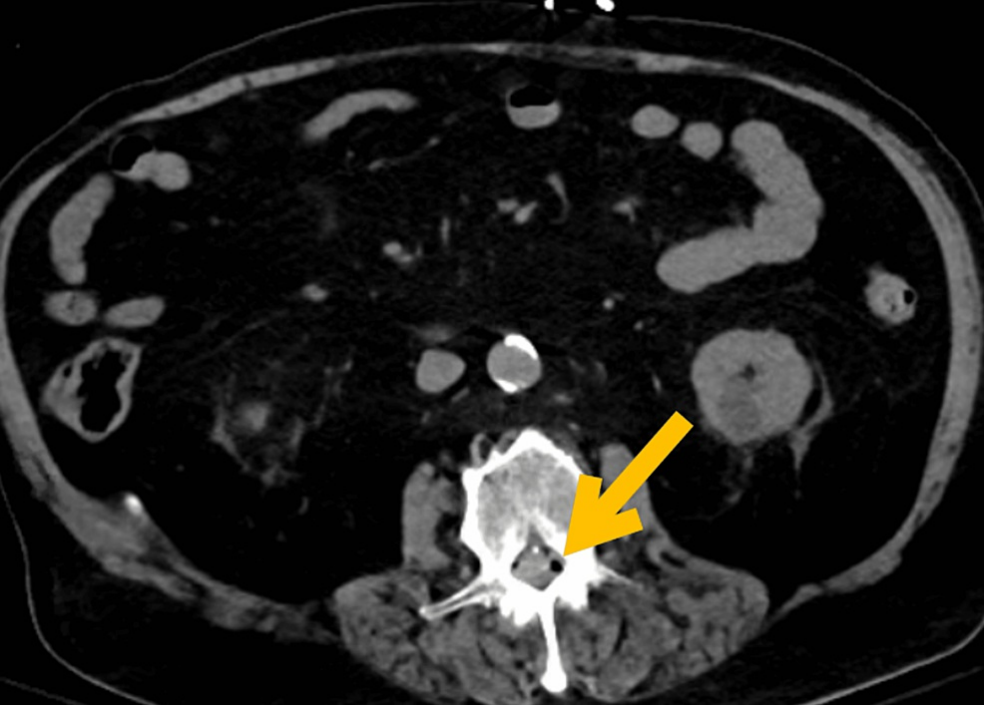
Non-contrast CT of the abdomen and pelvis (axial section through the lumbar spine). Air bubbles within the epidural space of the lumbar spinal canal (arrow). CT: computed tomography
